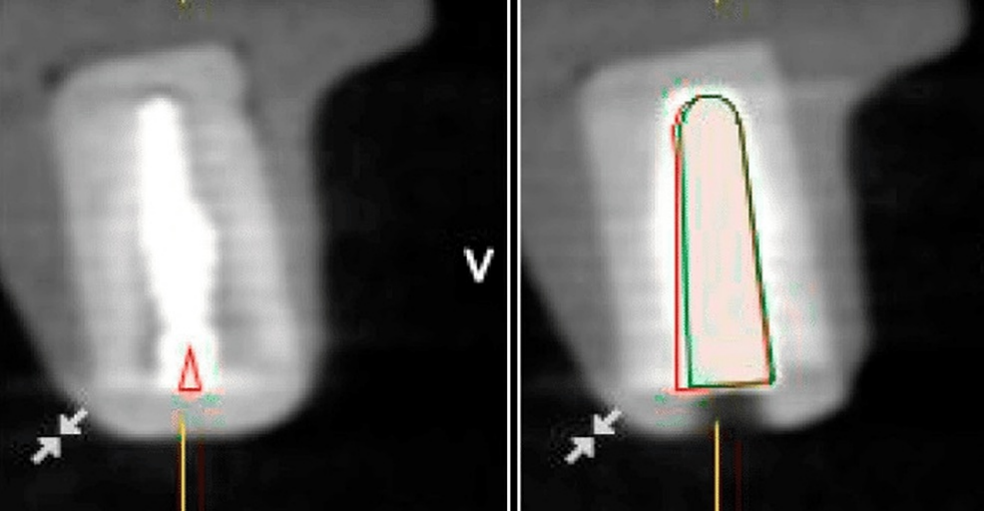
Conventional CBCT Scan of the Implant After Placement CBCT: cone-beam computed tomography
